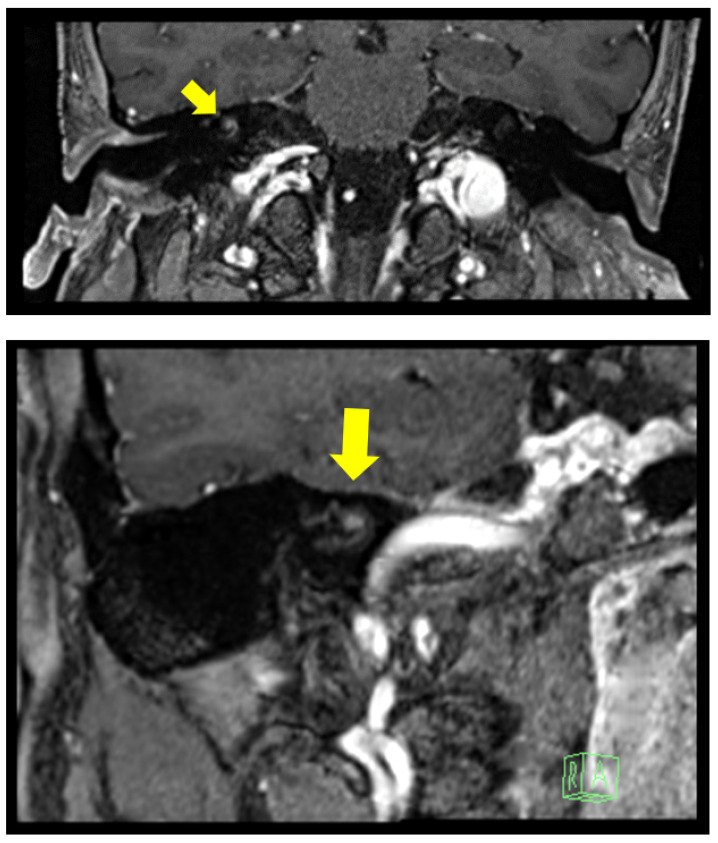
Clinical Case 2: 3 Tesla MRI with gadolinium showing a nodular enhancement, 2.7 mm in diameter, located on the right cochlear basal turn originating from the modiolus.
